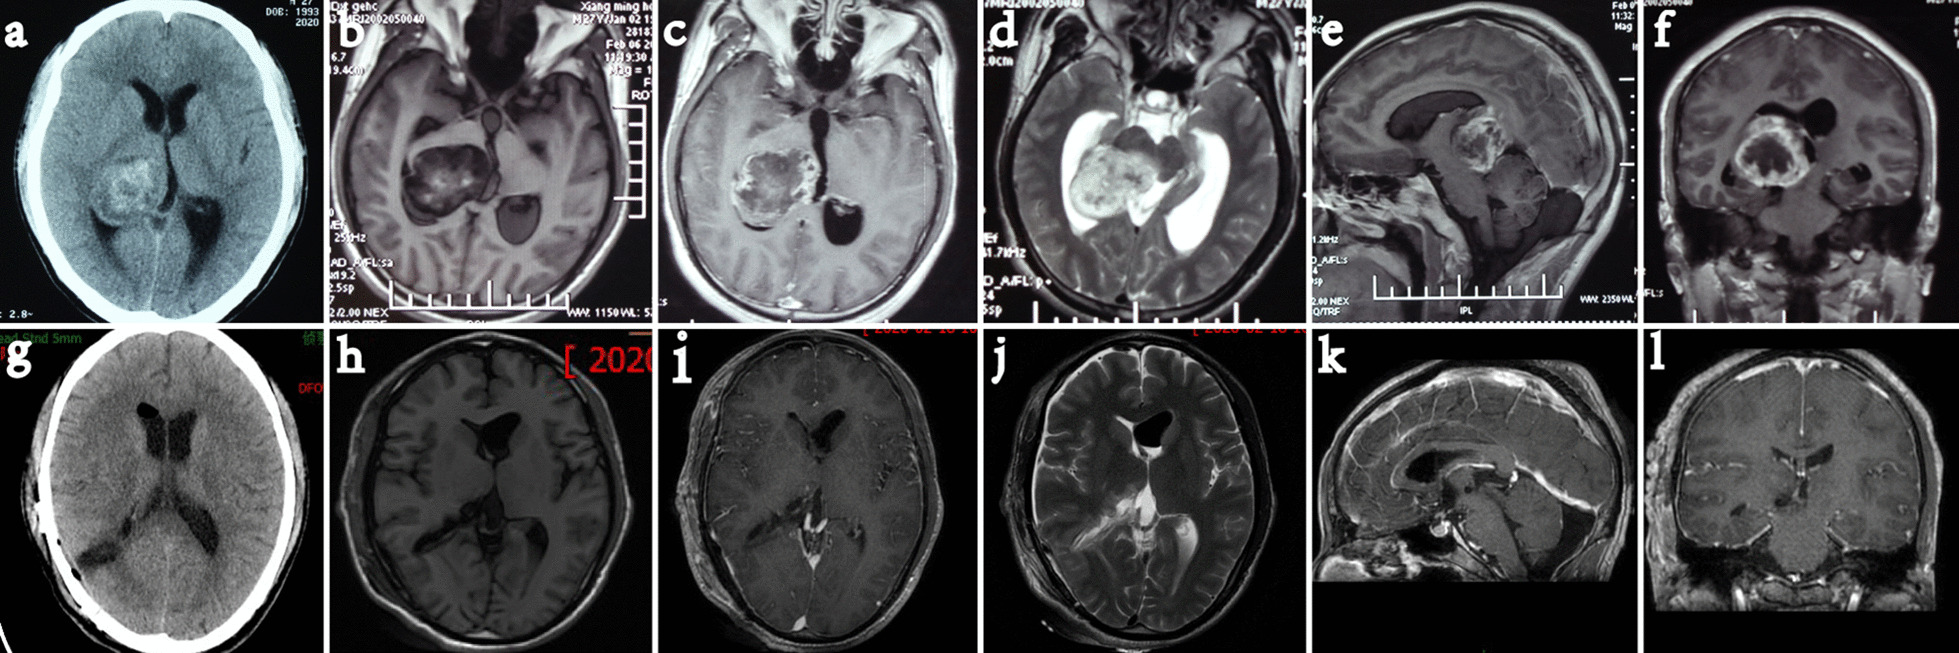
Case 3. Cranial CT and MRI revealed a unilateral thalamus located in the right thalamus. Preoperative CT and MRI (a–f). Postoperative CT and MRI confirmed total resection (g–l)
